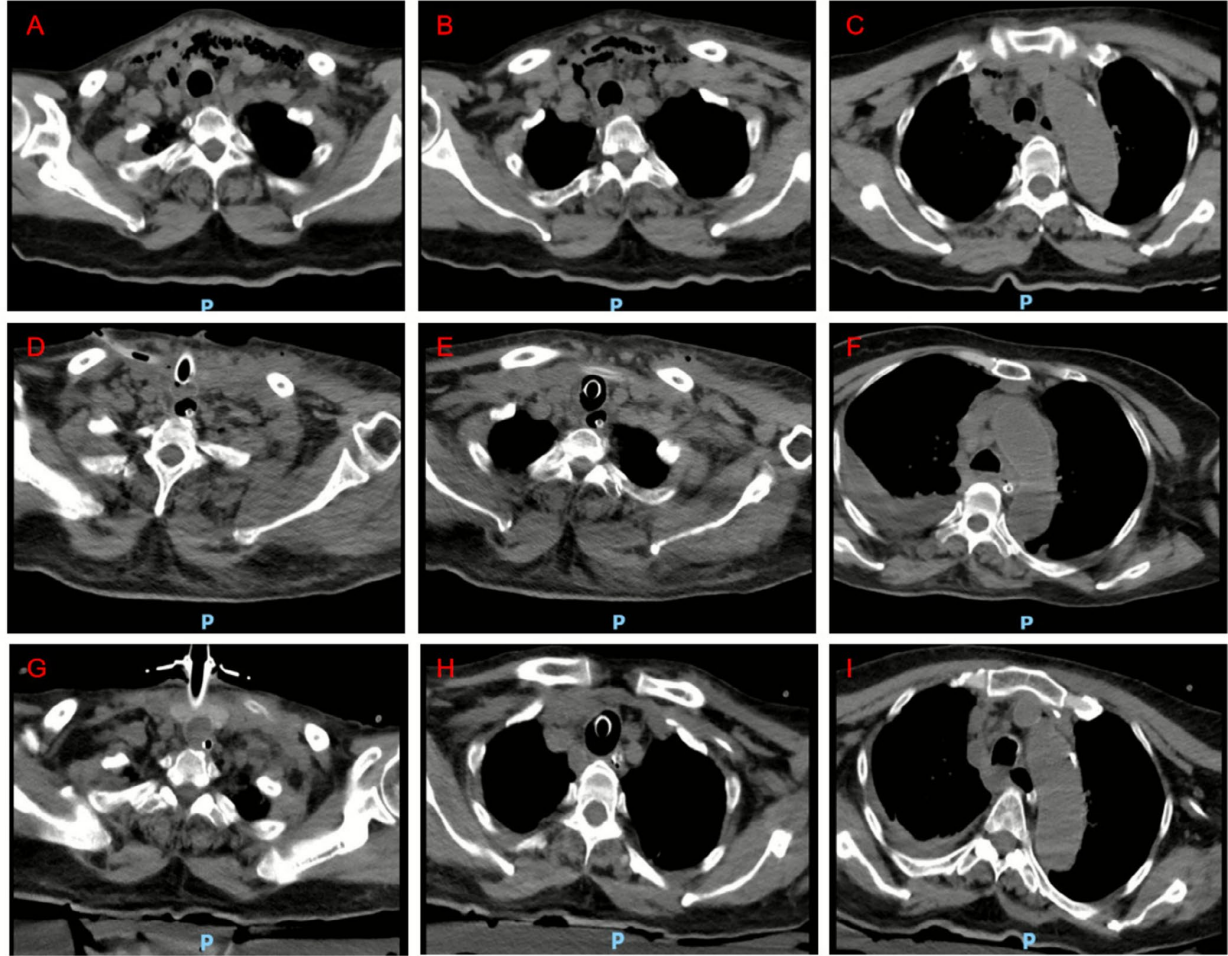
CT images acquired at different time points during the patient's hospitalization. (A–C) April 21; (D–F) May 13; (G–I) June 2.
